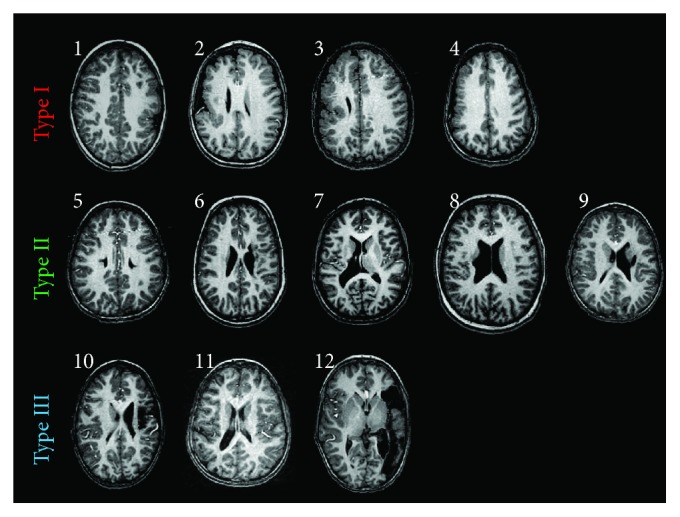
Representative slices of 3D T1-weighted images depicting the brain lesion of each UCP child. Numbers correspond to the ID of UCP children as reported in Table 1.
